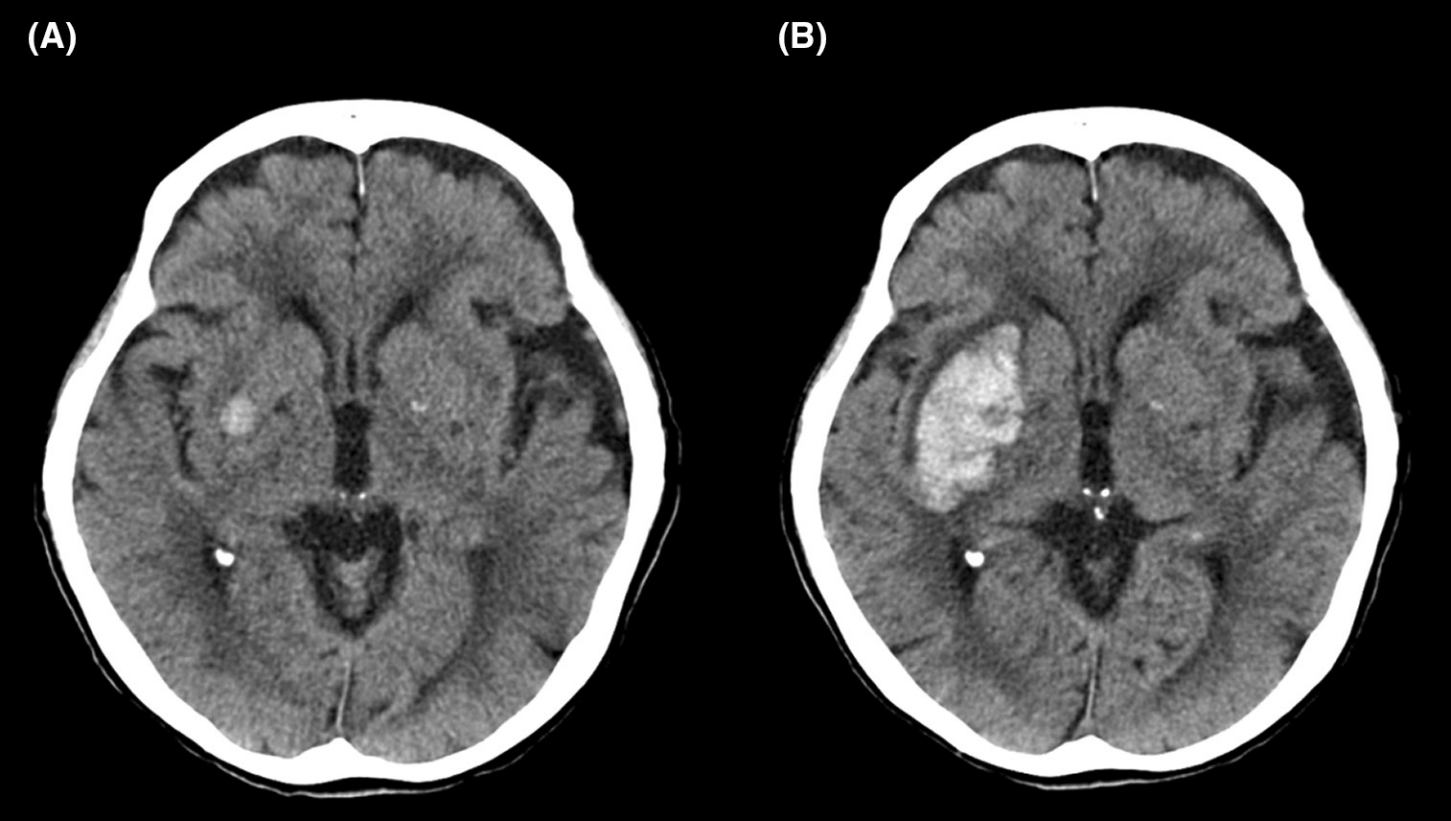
Computed tomography scan of the patient's head. A: An intracranial hemorrhage measuring 9 × 9 × 5 mm was revealed in the right basal ganglia on admission. B: The intracranial hemorrhage increased to 52 × 35 × 30 mm 3 h after admission
